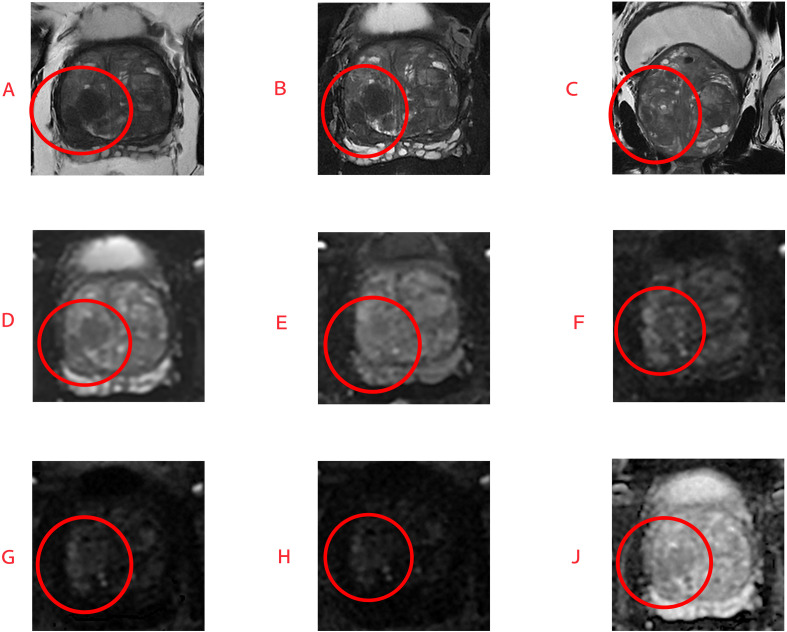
This is magnetic resonance imaging of a 60-year-old patient with benign prostatic hyperplasia. T2-weighted images (A–C) reveal a poorly defined low-signal lesion in the anterior transition zone; DWI sequences at b=0 (D) and b=800 (E) are also shown, and this lesion shows slightly increased signal intensity on the DWI sequence at b=1500 (F), while no significant signal enhancement is observed on sequences at b=2500 (G) and b=3000 (H). The ADC map is shown in (J). Subsequent biopsy revealed no evidence of prostate cancer, further indicating the diagnostic value of high b-value DWI for lesions in the prostate TZ, transition zone.
